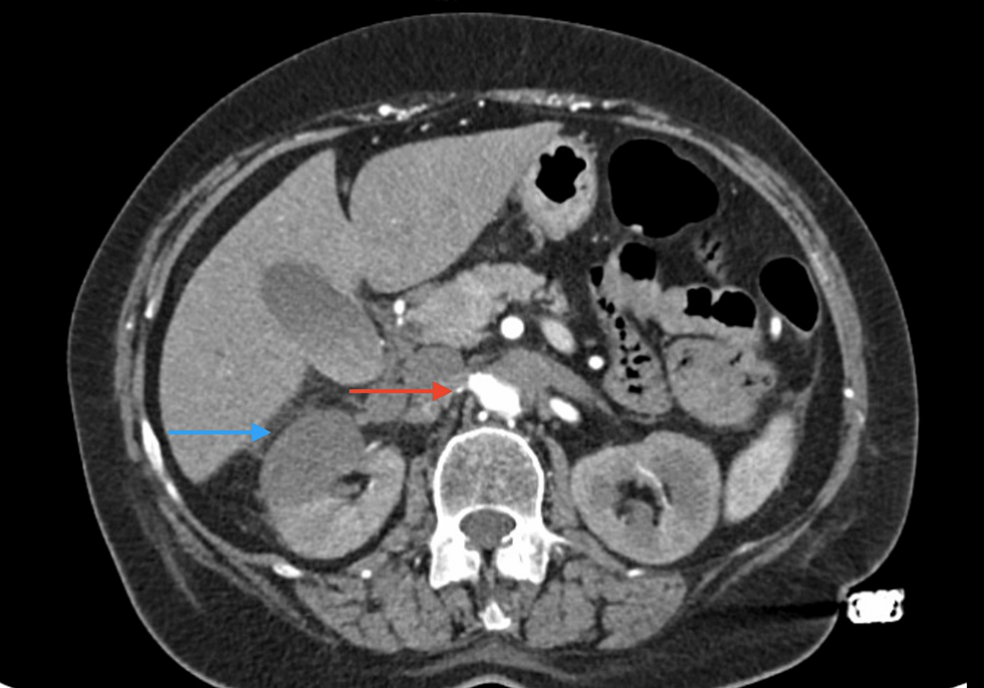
Abdominal CT angiography confirming an extensive opacification defect of the right renal artery (red arrow) and a parenchymal infarction (blue arrow), confirming the diagnosis of a recent renal infarction.
